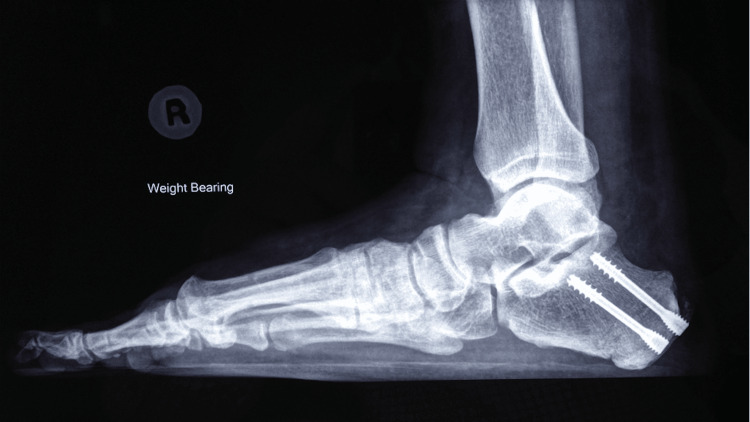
Post-operative weight-bearing lateral radiographs of the patient’s right foot.
